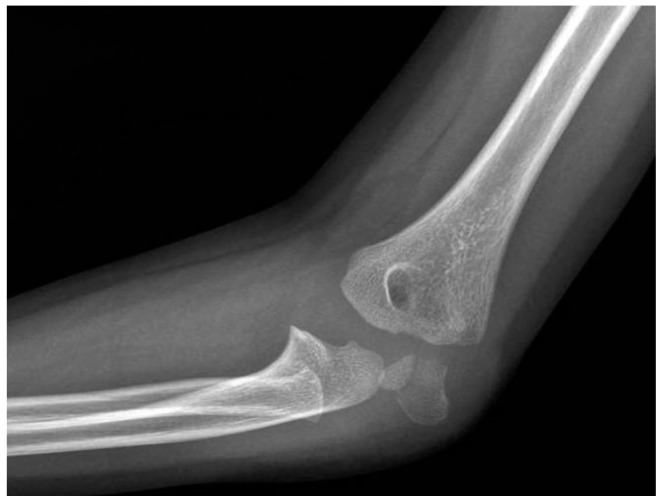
Pre-operative X-ray (lateral view).
